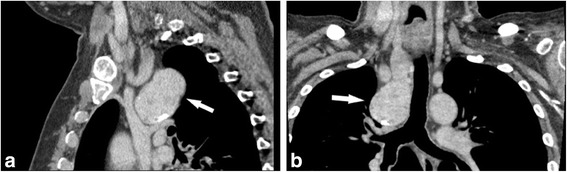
a Lateral view and b Frontal view of enhanced-CT scan of neck and chest showing the mediastinal mass (white arrow) and the mediastinal structures around
